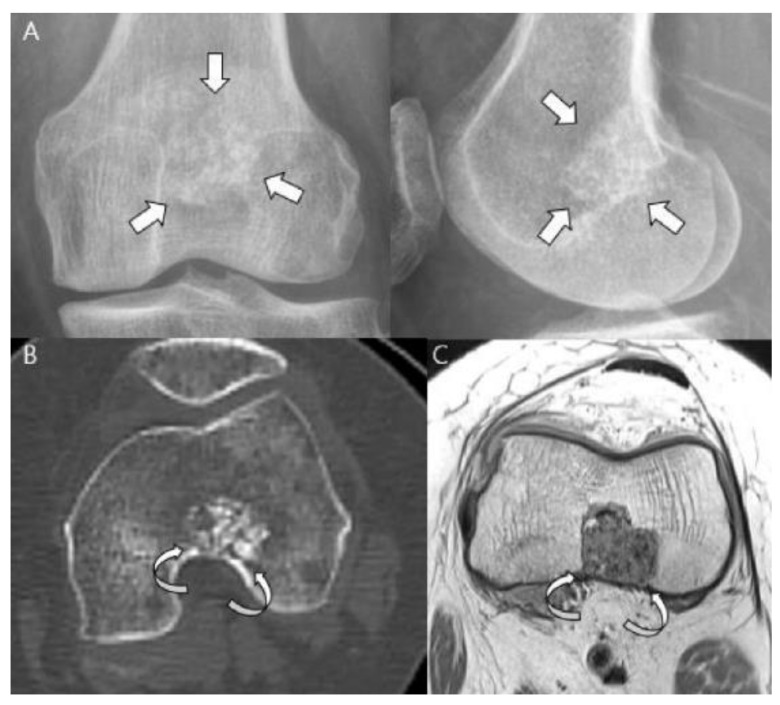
Atypical cartilaginous tumor of the distal femur in a 50-year-old woman. (A) Anteroposterior and lateral radiographs reveal a mixed lytic and sclerotic lesion in the distal femur (arrows) with typical ring-and-arc calcifications. (B) Computed tomography and (C) axial T2-weighted image demonstrate a lobulated chondroid tumor with deep endosteal scalloping (curved arrows) despite the small tumor size (1.7 cm).
